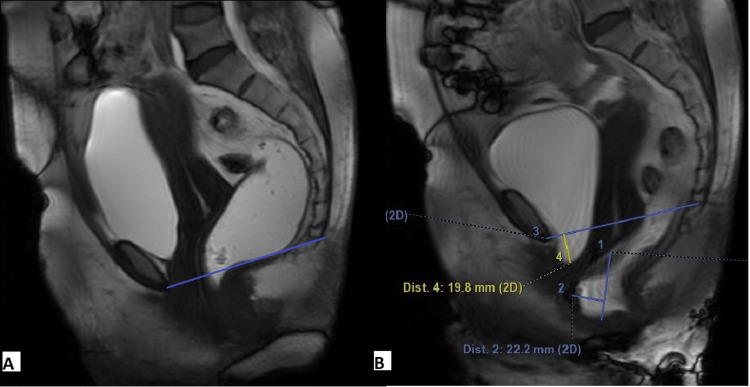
A 48-year-old female complained of stress incontinence and incomplete emptying of the rectum. Sagittal dynamic FIESTA MRI image in rest phase (A) reveals the bladder and anterior cervical lip are above the PCL. Sagittal dynamic FIESTA MRI image in strain phase (B) shows mild cystocele, mild uterine prolapse, and anterior rectocele. FIESTA: fast imaging employing steady-state acquisition; PCL: pubococcygeal line.
